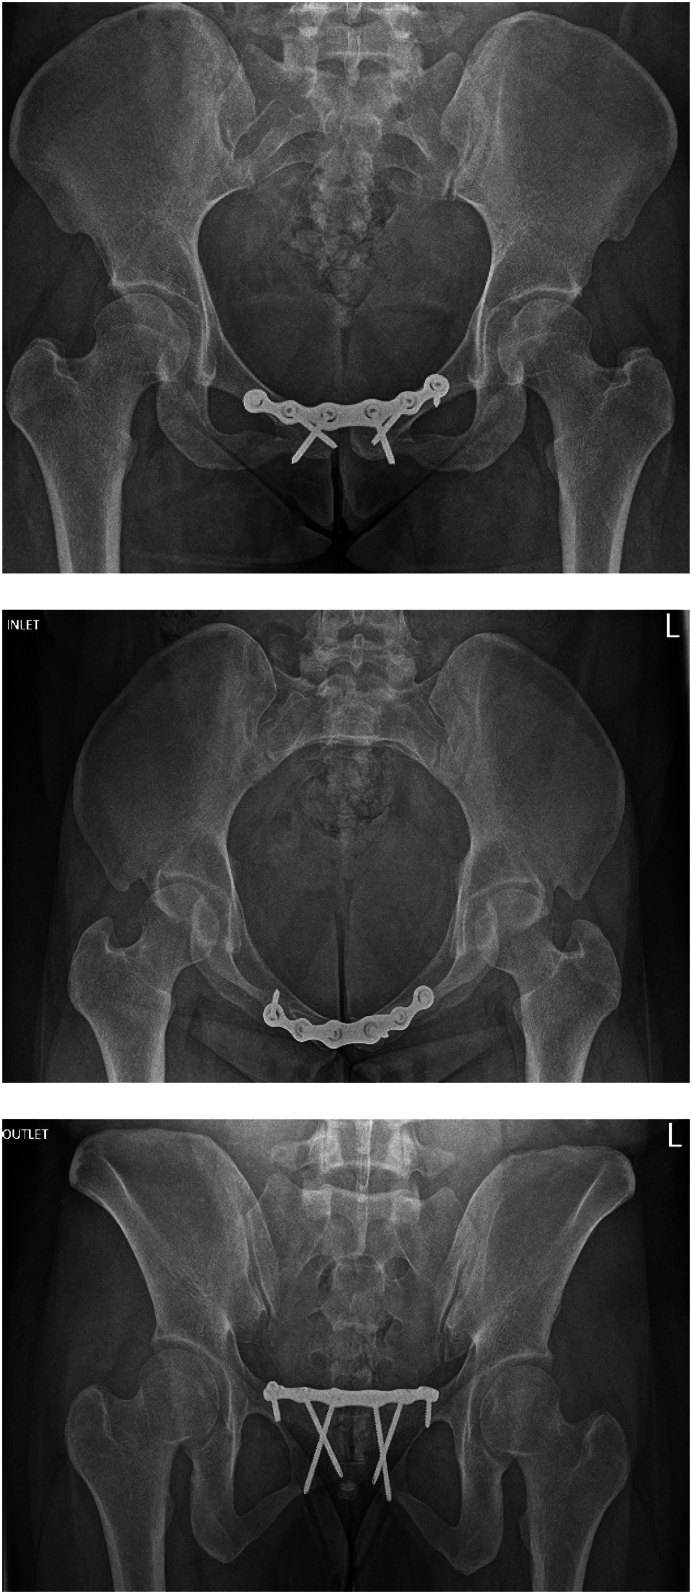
x-ray: an AP (1), inlet (2) and outlet (3) view of the pelvic.
